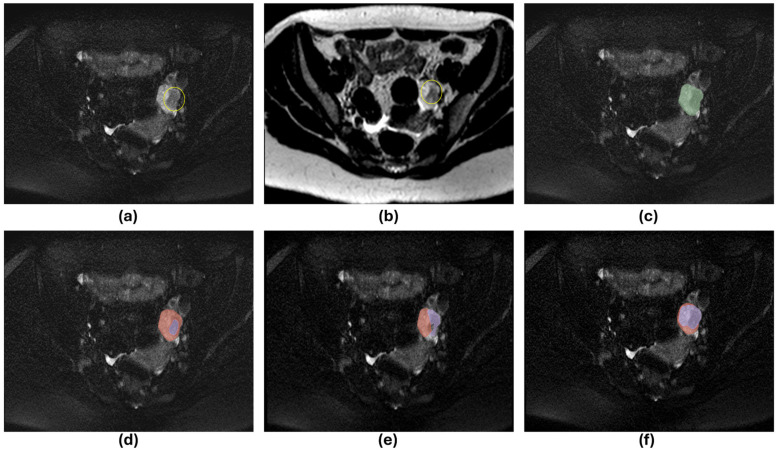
FS T2W and T2W MRI scans with FP cyst predicted by FS, T2W_FS_ + FS, and T2W + FS_T2W_ AI models on a subject from the in-house test cohort. (a) FS T2W MRI with visible cyst (yellow circle) with size <3 cm, (b) T2W MRI with visible cyst (yellow circle) with size <3 cm, (c) expert annotation on FS T2W MRI. The cyst was not contoured as the size of the cyst was less than 3 cm. (d) AI-predicted ovary (red) and cyst (purple) contours on the FS T2W scan by the T2W_FS_ + FS model. The T2W_FS_ + FS model is slightly under-segmenting the cyst. (e) AI-predicted ovary (red) and cyst (purple) contours on the FS T2W scan by the T2W + FS_T2W_ model. (f) AI-predicted ovary (red) and cyst (purple) contours on the FS T2W scan by the FS model. It is visible that the T2W + FS_T2W_ and FS models are over-segmenting the cysts and including the normal ovary region.
